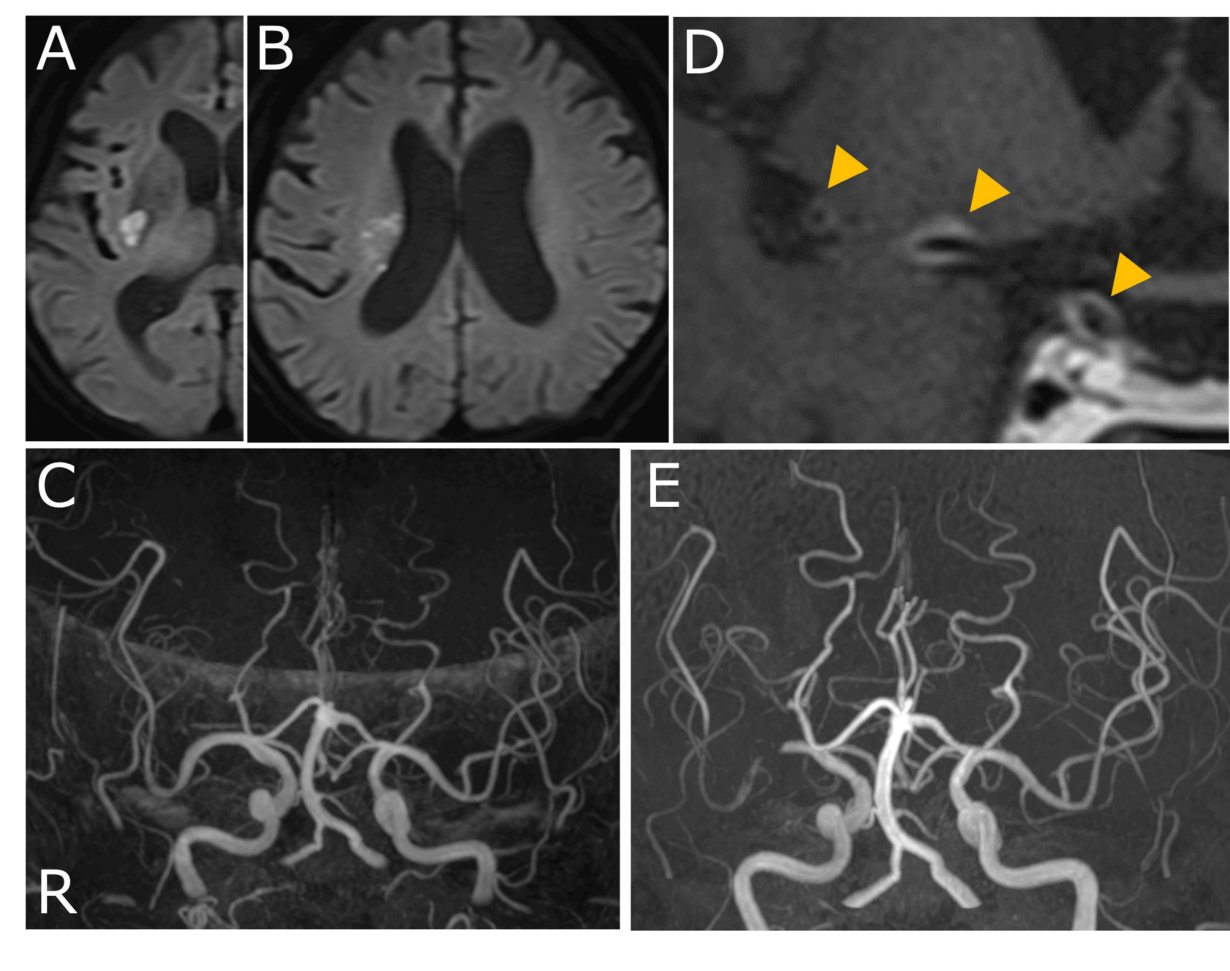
Brain MRI at initial assessment and disease progression. (A, B) Diffusion-weighted images on admission show a small region with diffusion restriction in the right putamen and blurred and small patchy lesions in the corona radiata on Day 45. (C) MRA showed no cerebral artery stenosis on Day 45. (D) A contrast-enhanced T1-weighted image reveals circumferential vessel wall thickening and gadolinium enhancement at the right ICA terminus and the distal portion of the right middle cerebral artery (MCA) (arrowheads) on Day 45. (E) MRA showed right severe MCA stenosis and reduced distal vessel intensity on Day 82.
